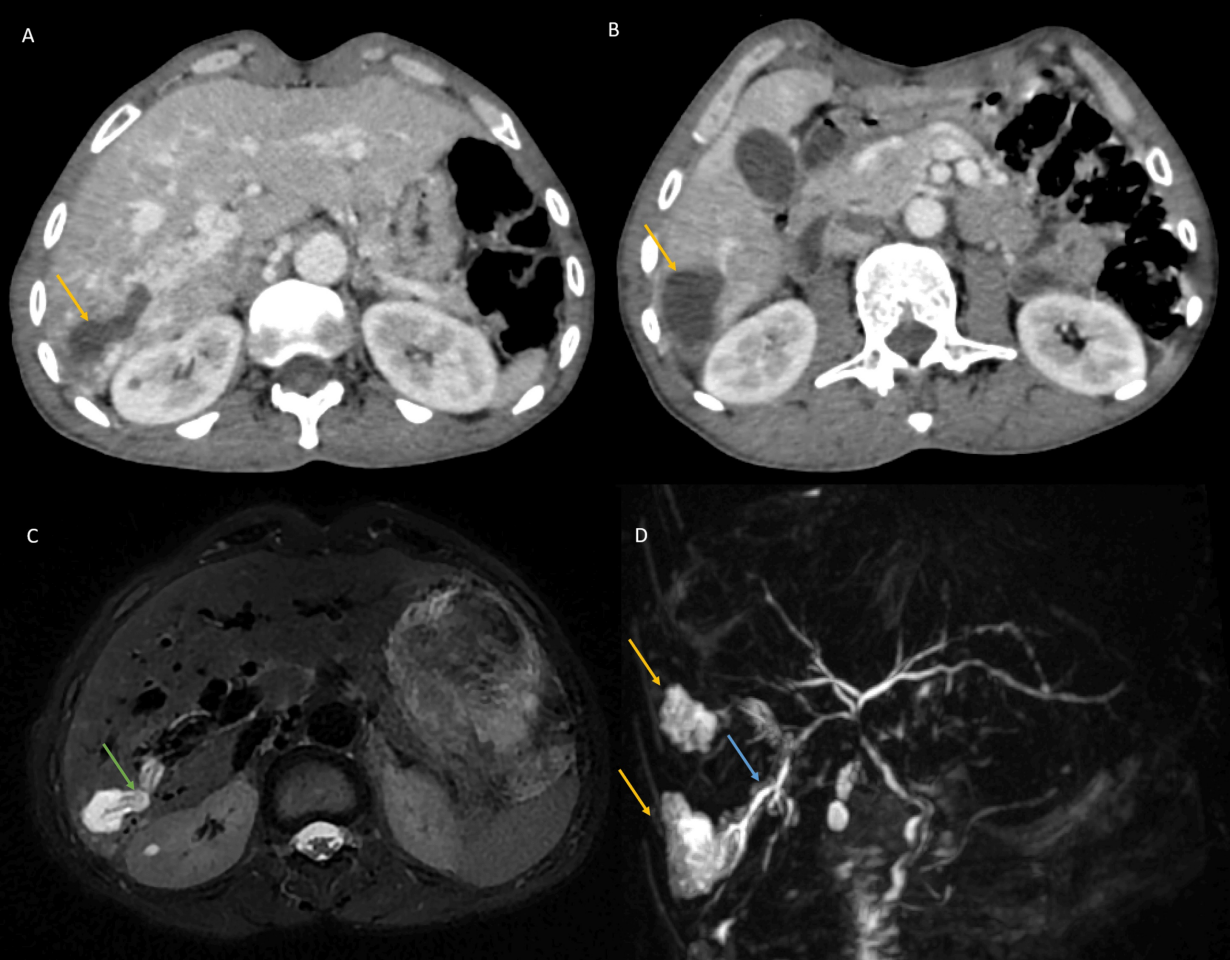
Axial portal phase CT-scan (A, B) and liver MRI in axial T2W FS (C) and coronal T2 RARE (D) showing biliary involvement in hereditary hemorrhagic telangiectasia There are cystic lesions in the VI and VII segments (yellow arrows), communicating with biliary trees (blue arrow), with fluid-fluid level (green arrow) and no enhancement after injection, suggestive of a bilomas. T2W FS: T2 weighted fat saturated; RARE: rapid acquisition with relaxation enhancement
